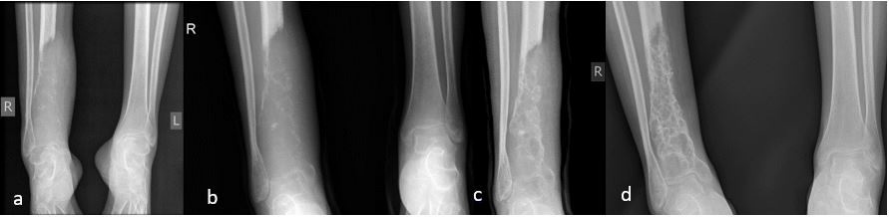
Right ankle radiographs showing (a) lytic lesion with soft tissue component in the distal right tibia with progressive bone formation in (b) after 1 cycle of chemotherapy (c) after 2 cycles of chemotherapy and (d) post radiotherapy and 4 cycles of chemotherapy.
